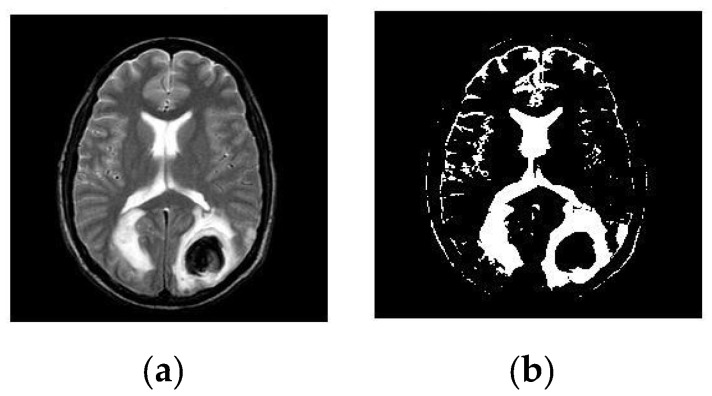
Here, image (a) is an input T-2 brain MRI image, whereas (b) is a segmented T-2 image. It is a Malignant Tumor according to the segmented picture.
